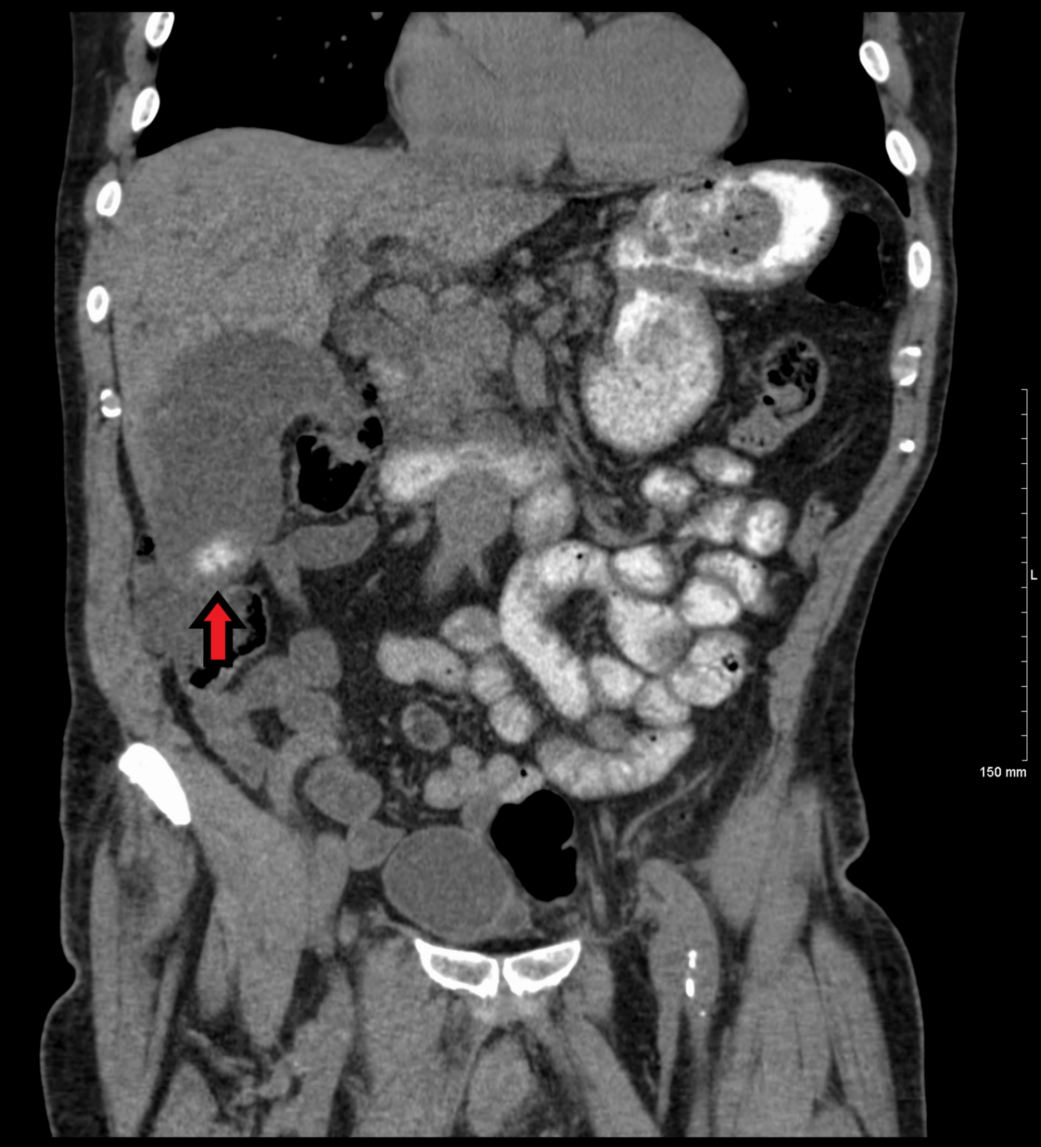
Coronal CT of the abdomen with oral contrast demonstrating a significantly dilated gallbladder. A 3 cm calcification is seen in the dependent portion of the fundus (arrow) CT - Computed Tomography
